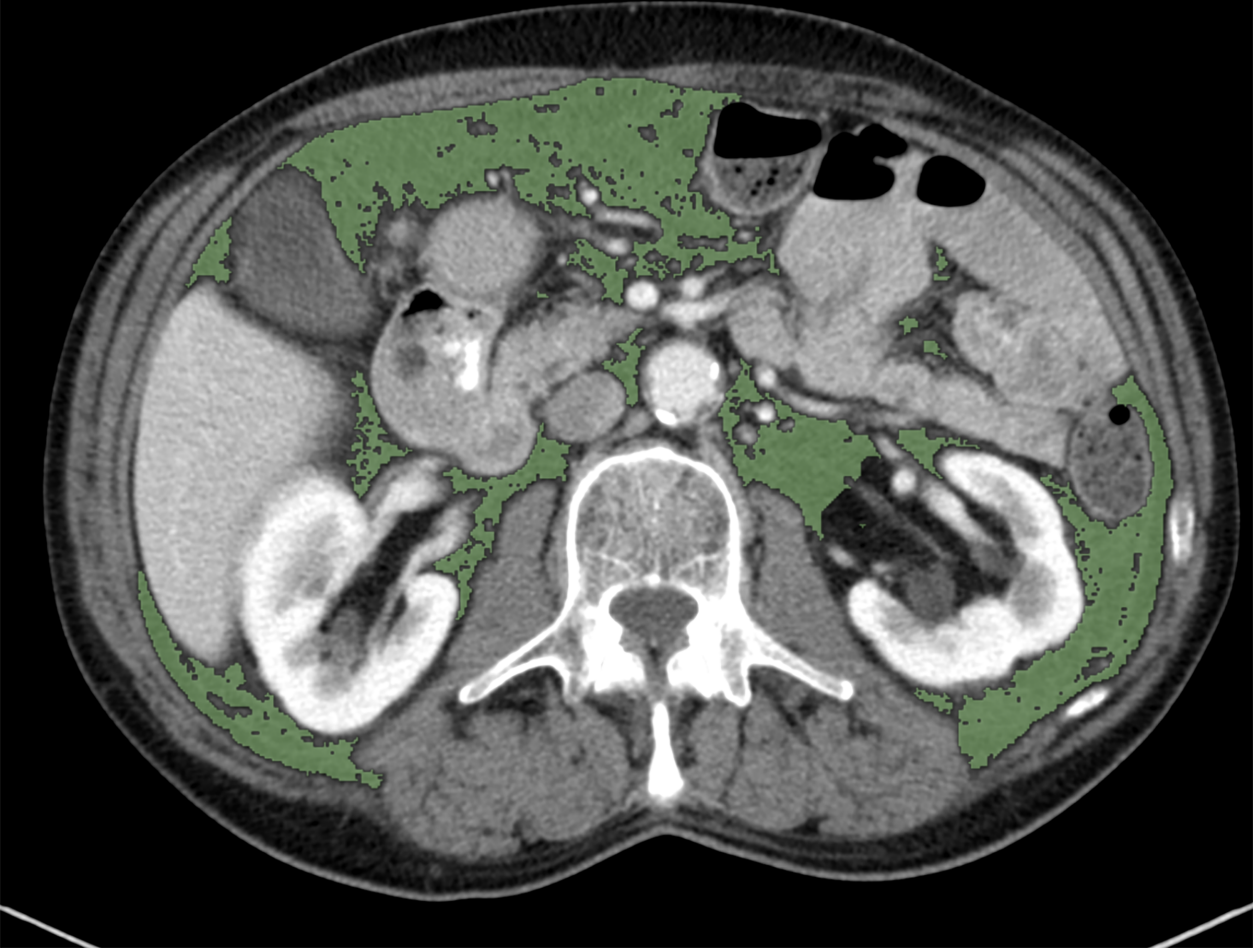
Measurement of VFA on the level of L3. From an axial CT image segmentation of visceral fat is performed in the range of -150 to -50 HU.
